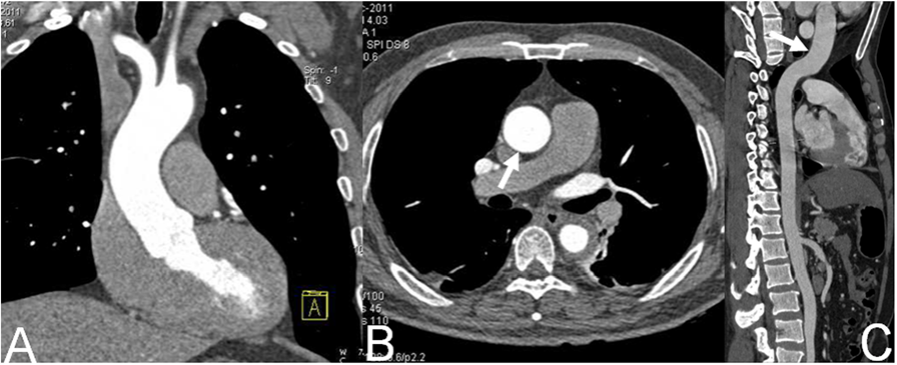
Dual-source CT imaging with high-pitch mode. Coronal MPR image (A, white arrow), axial original image (B, white arrow) and CPR image (C) show the wall of the entire ascending aorta is smooth without pulsation artifact.
